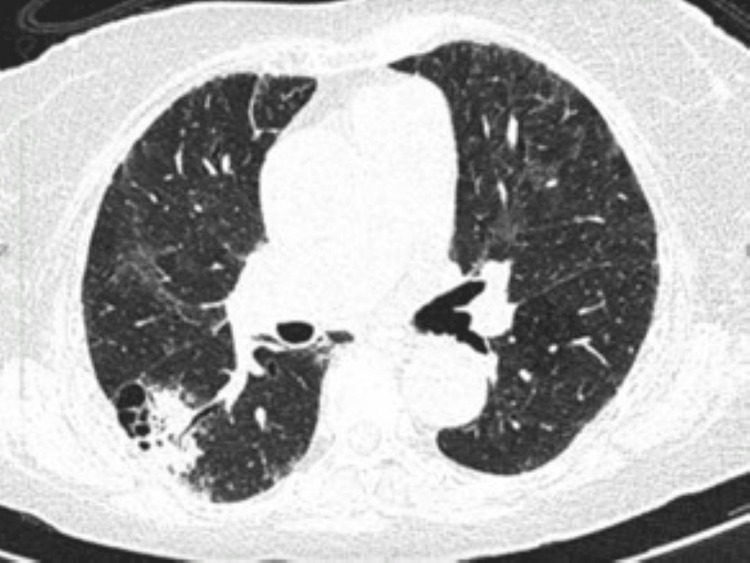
Computerized tomography (CT) of Case 2. A 4.2 cm macrocystic focus within the right lower lobe is associated with an area of opacification and air bronchograms, appearances are in keeping with a superadded infection.
